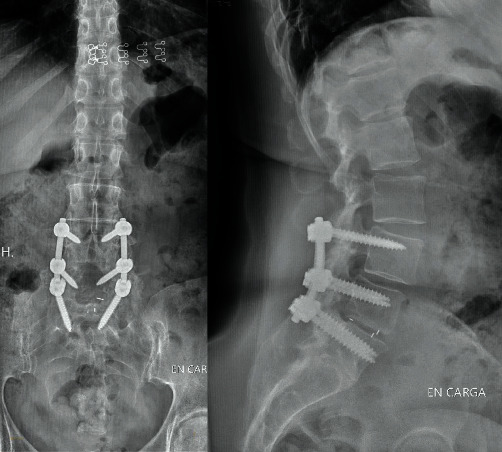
Standing radiographs in anterior-posterior and lateral projections. Postoperative study of the 5-year follow-up. We can observe the obtained fusion with and adequate position of the implants.
